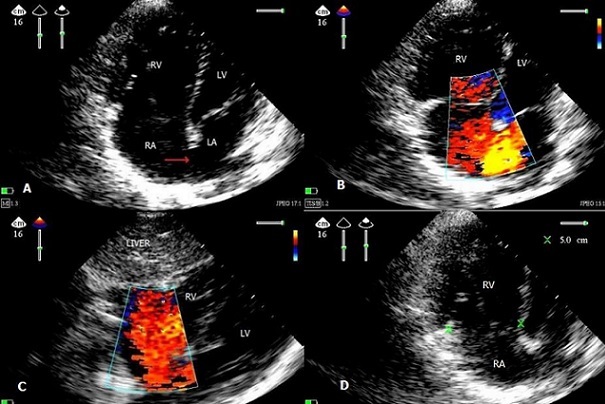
Ultrasound images A) apical four cavity 2d echocardiography image showing dilatation of right ventricular with rv / lv higher than 0.6 in diameter and surface, atrial septal defect with 3 cm type ostium secundum; B) apical four cavity with color doppler showing a left - right shunt; C) the subcostal window with color doppler showing the atrial septal defect with shunt; D) apical four cavity centred on the right ventricle showing the expansion of the ring tricuspid to 5 cm. (LA: left atrium, LV: left ventricle, RV: right ventricle, RA: right atrium, Red arrow: atrial septal defect)
